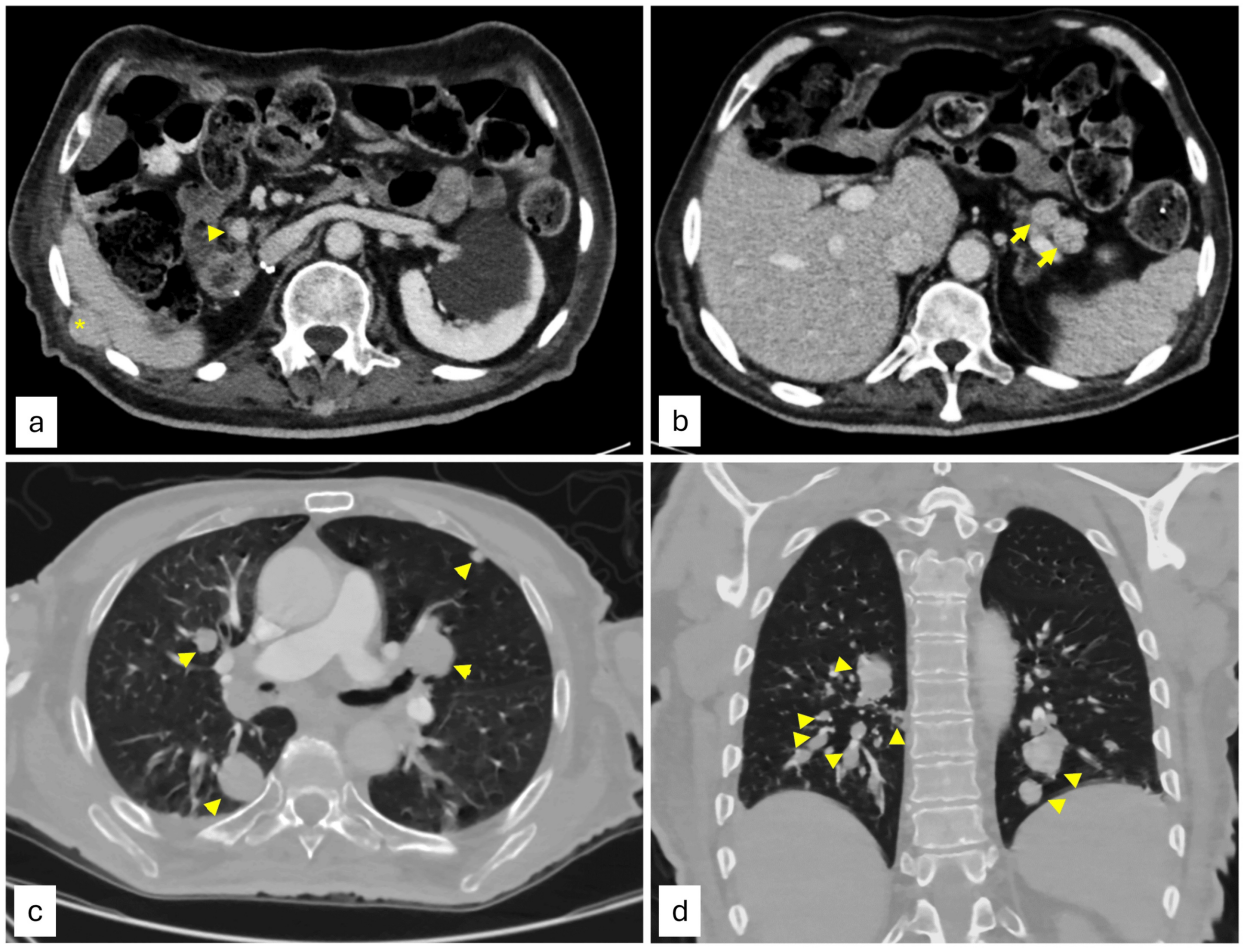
Contrast-enhanced CT scan images (a) & (b) Axial contrast enhanced CT scan images of the upper abdomen showing hyper-enhancing lesions involving the pancreatic head (arrowhead), right 10th intercostal space (*) and pancreatic tail (short arrow); CT scan images of the lungs in the (c) axial & (d) coronal planes, demonstrating multiple bilateral pulmonary nodules (short arrows).
